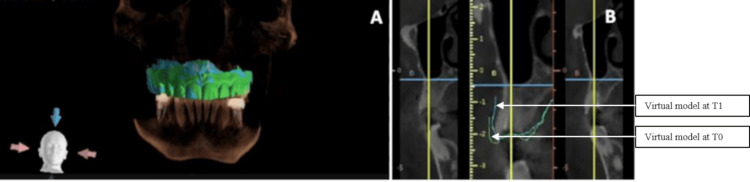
Virtual model superimposition with pre-extraction CBCT: (A) 3D view showing virtual model at T0 (green) and virtual model at T1 (blue), (B) sagittal view
